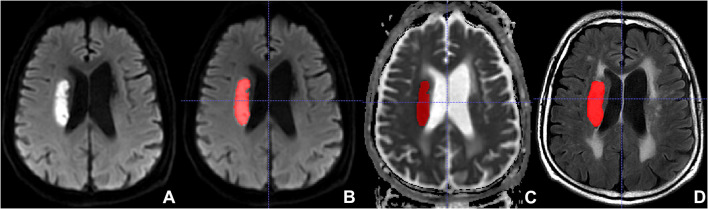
Lesion segmentation with the Insight Segmentation and Registration Toolkit-ANAP (ITK-SNAP). (A) Non-segmented diffusion-weighted imaging (DWI). (B) Segmented DWI. (C) Segmented apparent diffusion coefficient (ADC). (D) Segmented fluid-attenuated inversion recovery (FLAIR).
